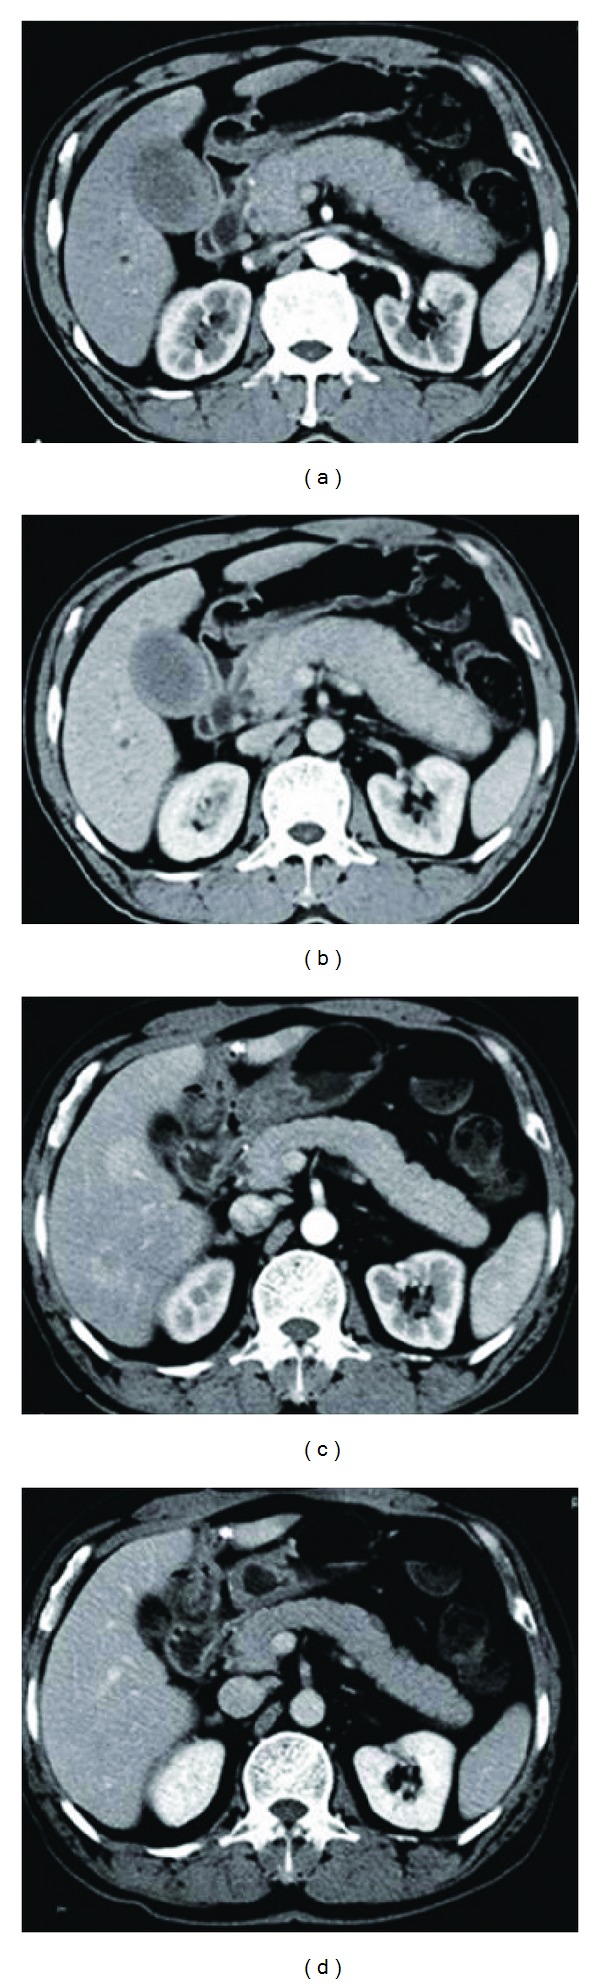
Imaging changes before and after steroid therapy in patient No. 6. Pretreatment: the arterial phase (a) and venous phase (b) of contrast-enhanced computed tomography both revealed a diffusely swollen pancreas with a “capsule-like rim” and “sausage-like” appearance. After internal drainage and a 3-month steroid therapy, the arterial phase (c) and venous phase (d) of contrast-enhanced showed that the swollen status of pancreas had been obviously reduced.
